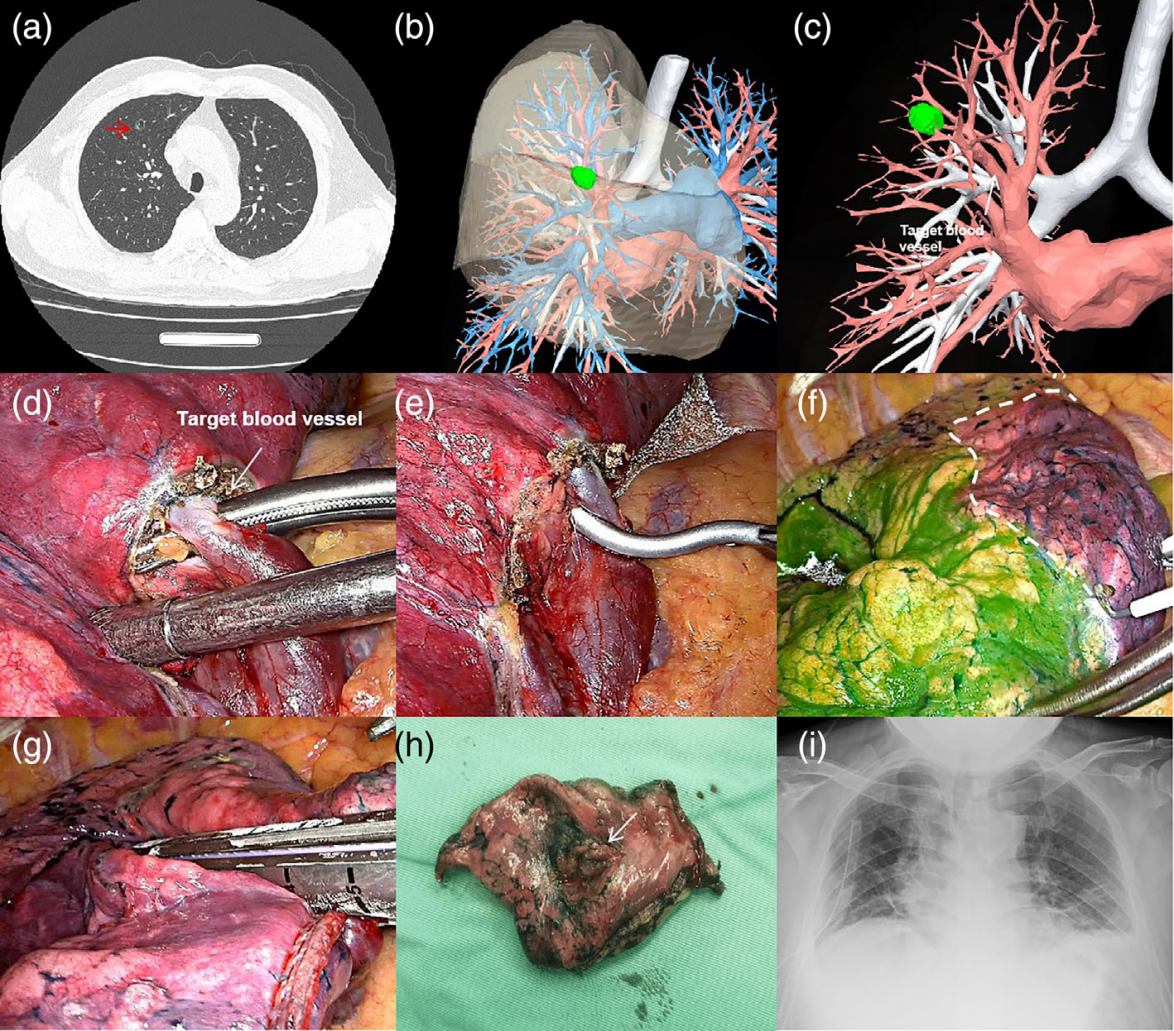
(a) Chest computed tomography (CT) examination revealed the precise localization of the nodule in the anterior segment of the right upper lobe of the lung. (b,c) The target vessel within the region where the nodules were located was identified and marked using three‐dimensional computed tomography bronchial angiography (3D‐CTBA) prior to the surgical procedure. (d,e) During the surgical procedure, the target vessel was dissected and temporarily occluded using noninvasive vascular clamps. (f,g) Following the intravenous injection of indocyanine green (ICG), a well‐defined resection boundary was observed. Subsequently, the target area was completely resected using endoscopic staplers, ensuring thorough removal of the intended tissue. (h,i) The nodule was successfully excised, and the resection margin was deemed adequate. Postoperative chest radiographs showed favorable recovery, indicating a positive outcome following the surgical procedure.
